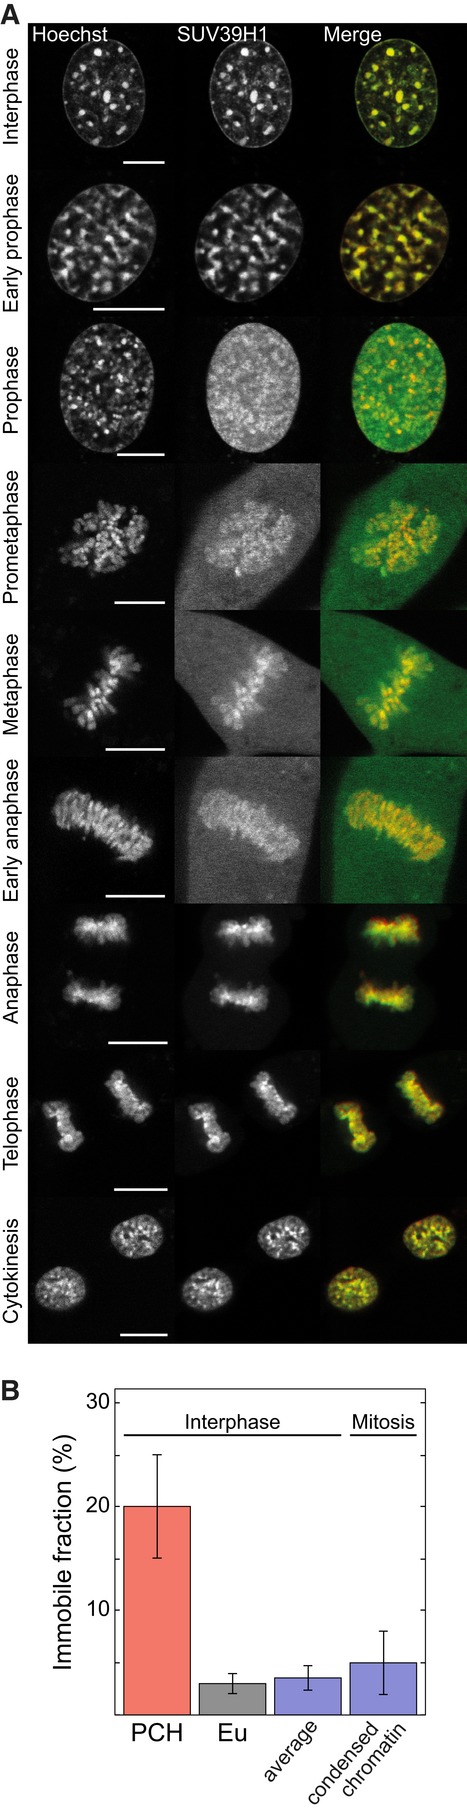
Chromatin bookmarking by SUV39H1 during the complete cell cycle Nuclear distribution of SUV39H1-GFP in living cells during different cell cycle phases.
